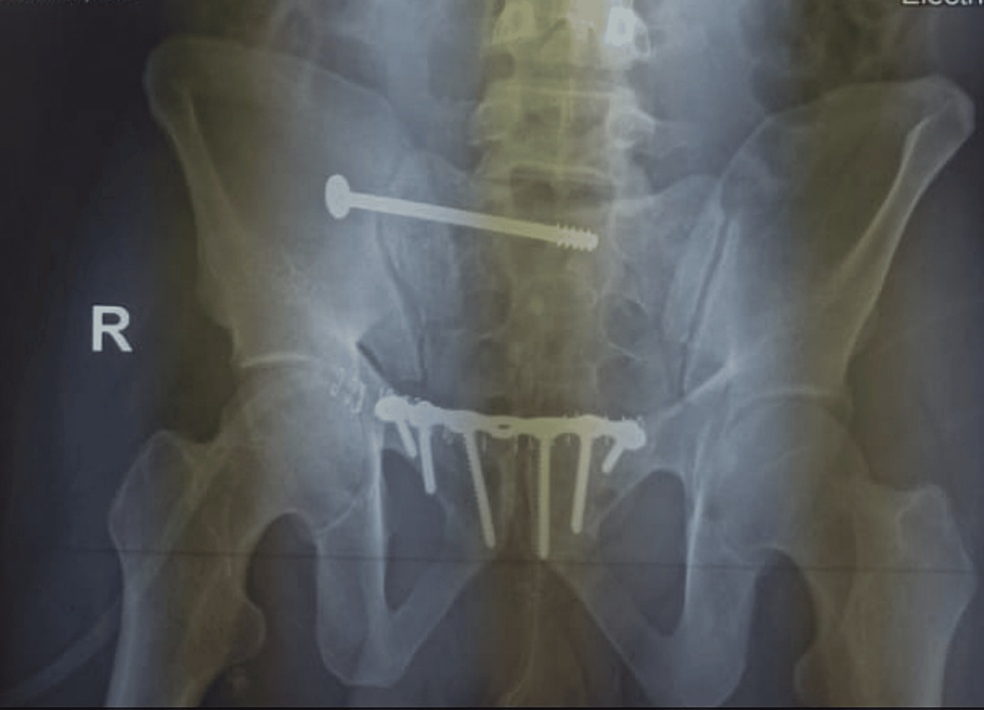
Postoperative X-ray outlet view of the pelvis showing excellent reduction of the sacral fracture using iliosacral screw fixation above the S1 foramen with fixation of the anterior pelvic ring injury using symphysis plating Image Credit: First Author
